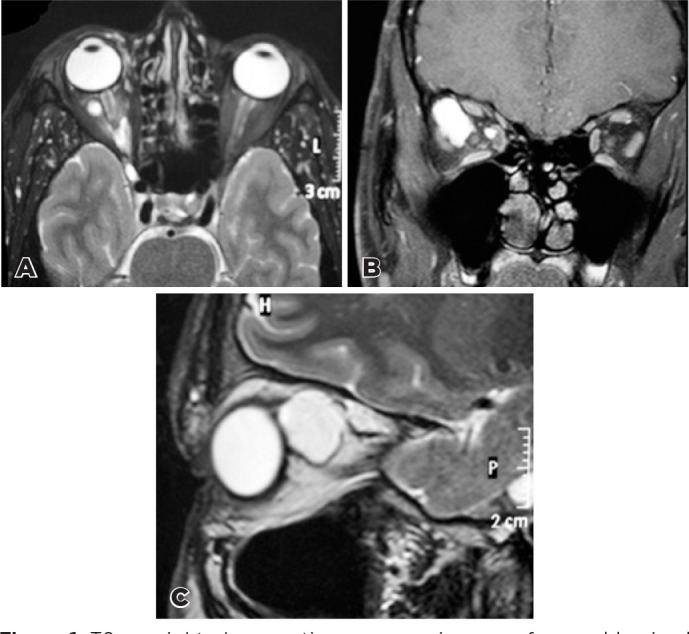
T2 - weighted magnetic resonance images of normal lacrimal tissue
